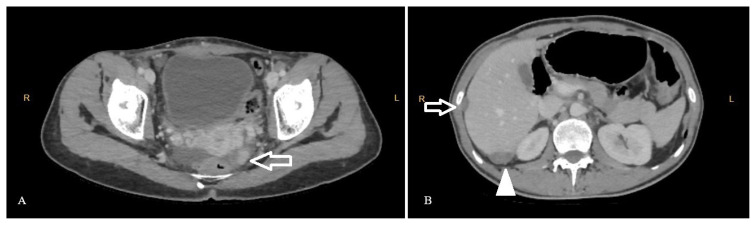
Axial view of the patient’s abdominal CT after primary surgery and chemotherapy. Contrast enhancing nodular implants are seen in the left pelvic peritoneum (Figure A, arrow). Figure B shows a 13-mm hypodense implant with punctuate calcifications causing indentation between liver segments 5 and 6 (arrow) and a 25-mm heterogenous hypodense lesion with calcifications on Morrison’s pouch (arrowhead).
